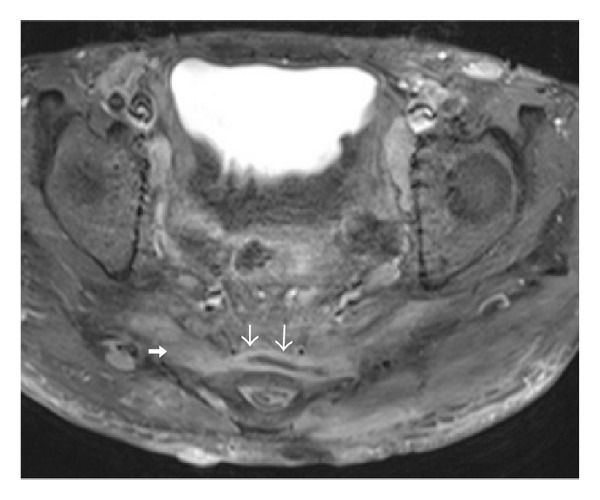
Axial T1-weighted fat-suppressed image postcontrast shows a well-defined rim-enhancing collection (white arrows) at the anterior perisacral space compatible with abscess, as well as abnormal marrow enhancement (thick arrow) in the sacrum consistent with osteomyelitis.
